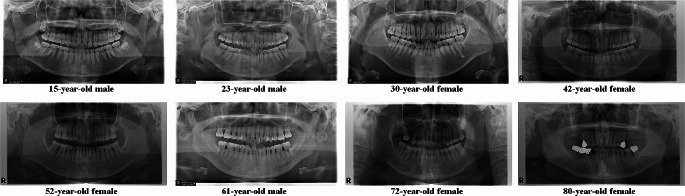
Examples of panoramic radiographs of males or females aged 15–80 years
